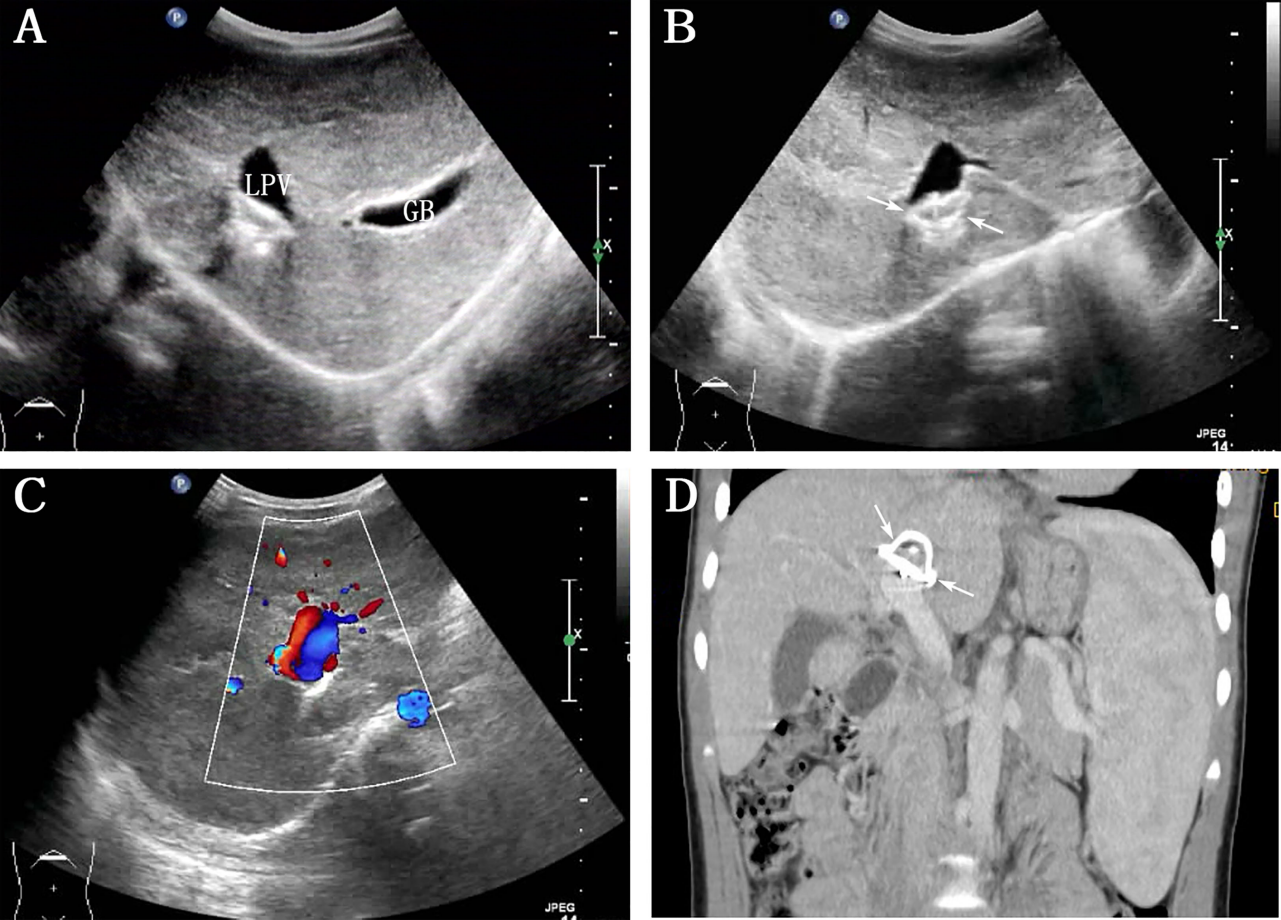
Abdominal ultrasound and contrast-enhanced computed tomography (CT) reexamination were performed 3 years after the interventional therapy. (A) Grayscale indicates the complete disappearance of multiple intrahepatic nodulars (LPV: left portal vein, GB: gall bladder); (B) hyperechogenicity between LPV and inferior vena cava (arrows); (C) color Doppler ultrasound image showed bidirectional venous waves in the intraportal spectrum; (D) contrast-enhanced CT on this reconstructed coronal view showed annular metal density shadows in the hilum of the liver (arrows).
